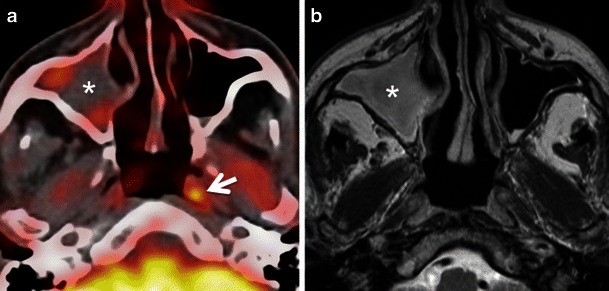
a Axial PET/CT image reveals asymmetrical FDG uptake in the nasopharynx (*arrow*) in a patient with SCC of the floor of the mouth. Opacification of the right maxillary sinus due to mucous retention (*asterisk*). b Axial T2-weighted MR image at the same level depicts symmetrical lymphoid tissue within the nasopharynx without underlying pathology. Endoscopy confirmed absence of tumour. Mucous retention in the right maxillary sinus (*asterisk*)
